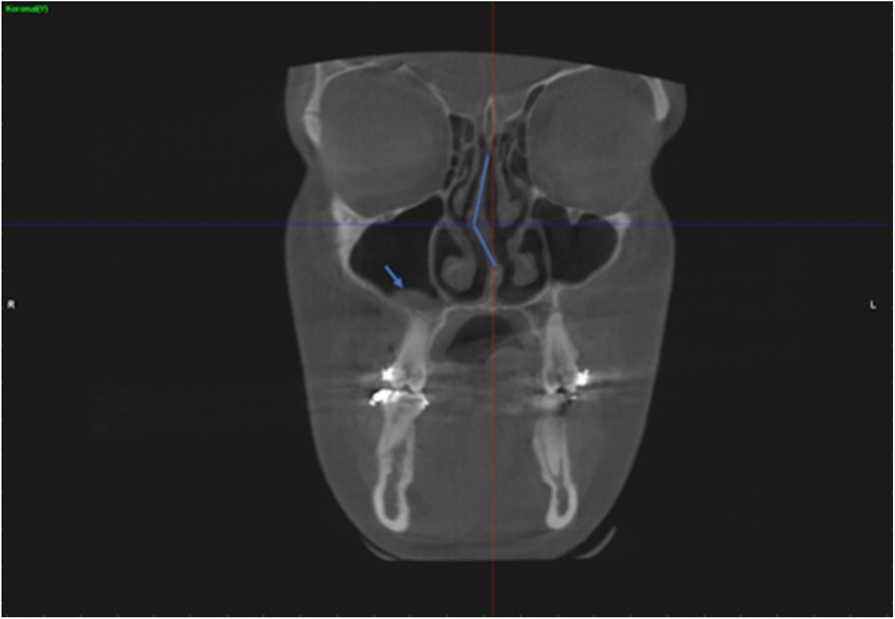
Determination of the coexistence of maxillary sinus pathology and nasal septum deviation in the coronal CBCT images
